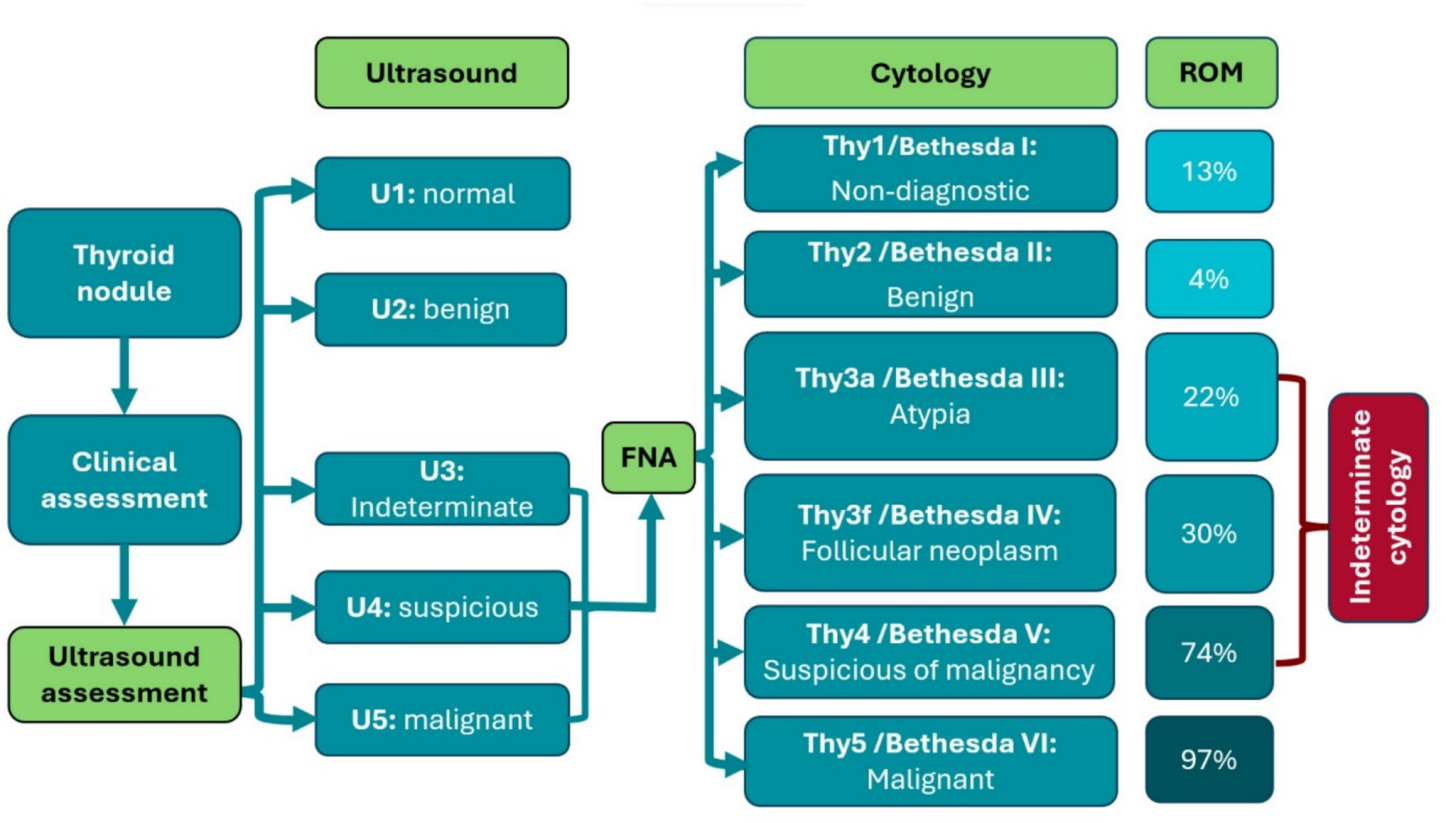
Summary of the clinical diagnostic pathway for thyroid nodules. After clinical assessment, patients undergo an ultrasound scan. Nodules with suspicious ultrasound features U3-U5 are subjected to fine needle aspiration (FNA) cytology (FNAC). Each cytological category is associated with a risk of malignancy (ROM), as defined by the Royal College of Pathologists in the UK and/or the 2023 Bethesda System for Reporting Thyroid Cytopathology [5]
